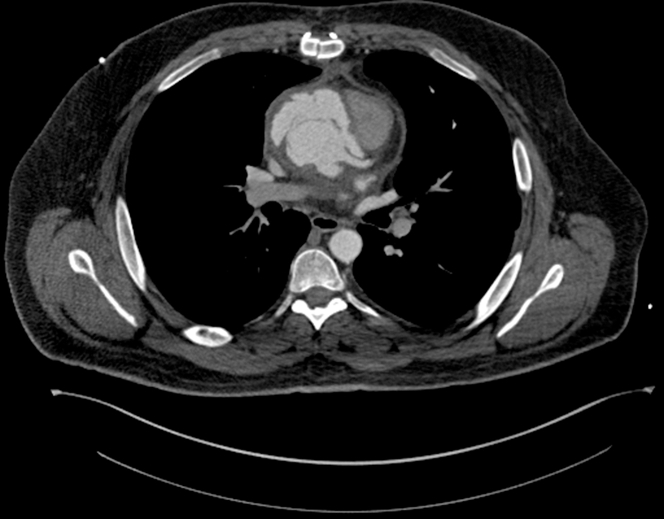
Axial Computed Tomography Chest View Showing Sinuses of Valsalva Pseudoaneurysm axial view of the computed tomography of the chest demonstrating contour abnormality involving the sinuses of Valsalva extending from the aortic root consistent with pseudoaneurysm.
